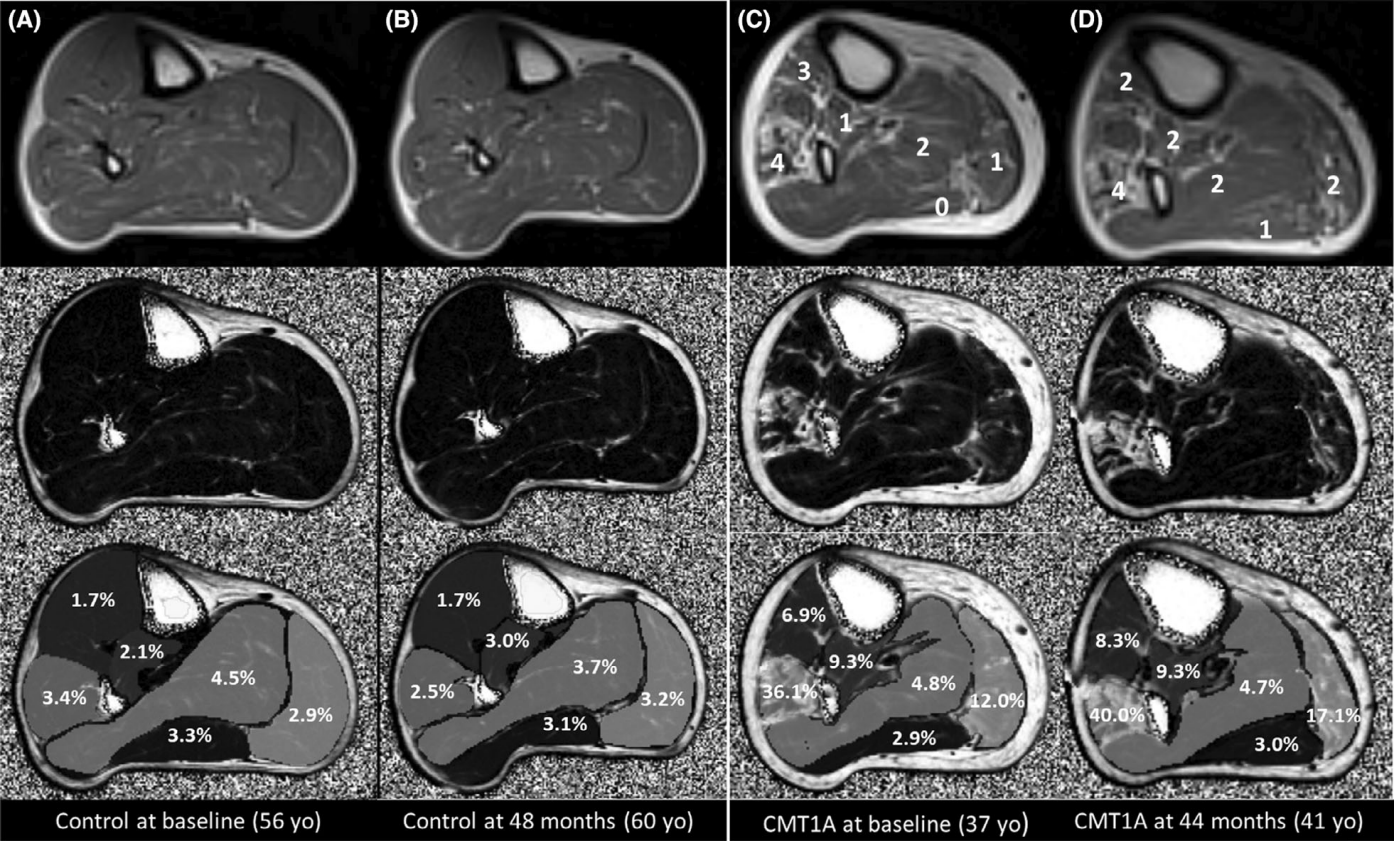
Example images. Axial MRI images of the right calf in a control at baseline (A) and last follow‐up (B), and patient at baseline (C) and last follow‐up (D). Top row: T1‐weighted images with modified Mercuri grades for the patient superimposed (based on whole muscle volume, all control muscles scored 0 on blinded analysis). Middle and bottom rows: Dixon Fat fraction maps (grayscale: 0%–100%ff) with muscle regions of interest and calculated mean fat fractions superimposed on bottom row. The control subject has normal fat fraction at baseline and does not significantly change over 4 years. The CMT1A patient shows variable fat fraction, highest in peroneus longus which increases in three muscles over 44‐month follow‐up.
